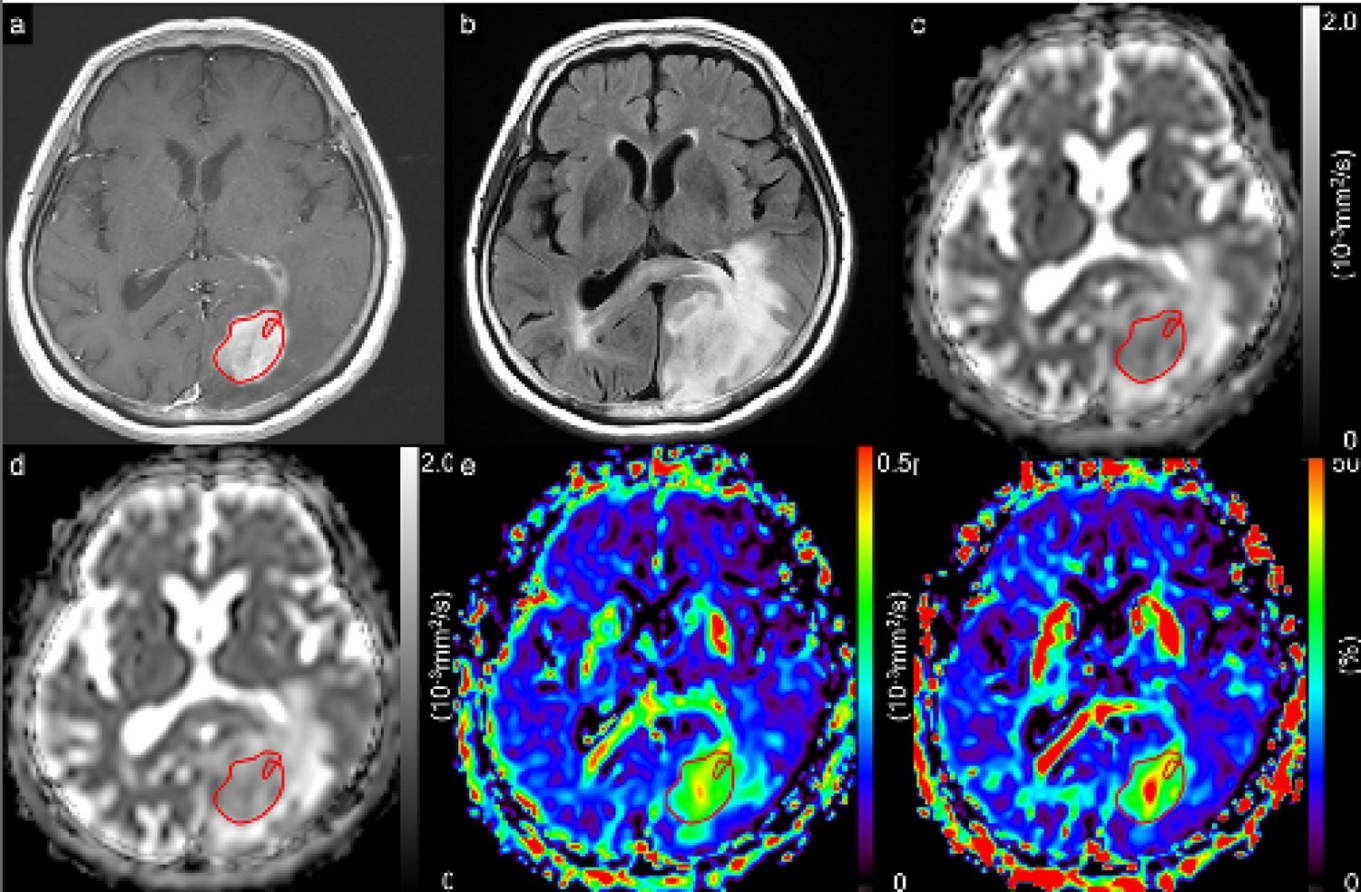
A 70-year-old female patient with glioblastoma, *isocitrate dehydrogenase*-wildtype, CNS WHO grade 4. A contrast-enhanced T1-weighted image with a ROI (solid line) (a). A fluid-attenuated inversion recovery image (b). ADC maps derived from PGSE DWI at a Δ_eff_ of 44.5 ms (c) and from OGSE DWI at a Δ_eff_ of 7.1 ms (d). Maps of cADC (e) and rcADC (f) between PGSE and OGSE. High cADC and rcADC levels in the tumor were observed. The mean ADC_44.5ms_, ADC_7.1ms_, cADC, and rcADC values are 0.988 × 10^− 3^ mm^2^/s, 1.118 × 10^− 3^ mm^2^/s, 0.130 × 10^− 3^ mm^2^/s, and 13.9%, respectively *Δ*_*eff*_ effective diffusion time, *ADC* apparent diffusion coefficient, *cADC* apparent diffusion coefficient change, *DWI* diffusion-weighted imaging, *OGSE* oscillating-gradient spin-echo, *PGSE* pulsed-gradient spin-echo, *rcADC* relative apparent diffusion coefficient change
